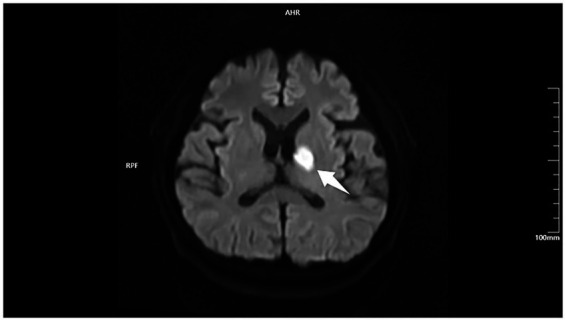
Admission brain diffusion-weighted imaging (DWI). Axial image shows an acute infarct in the left basal ganglia and periventricular region, presenting as marked hyperintensity (arrow). This lesion correlates with the patient’s right-sided hemiparesis, consistent with the imaging diagnosis of acute ischemic stroke.
